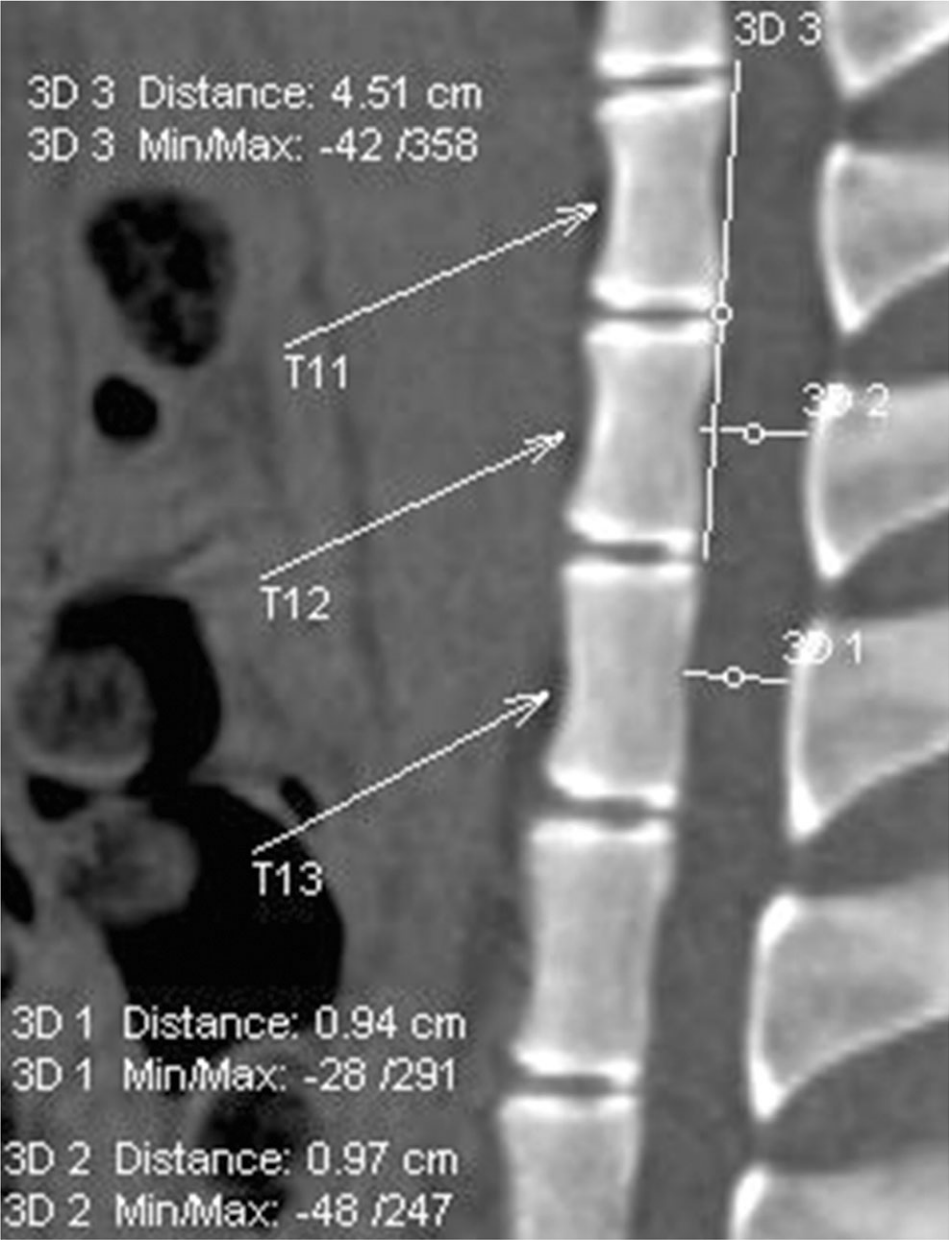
Initial computed tomography (CT) scan with measurements of the dimensions of the spinal cord length and diameter of the area of interest later for spinal cord balloon compression. The length of the spinal cord from thoracic (T) spinal cord level T11 to T13 is 4.51 cm. The diameter at T12 is 0.97 cm and at T13 0.94 cm
